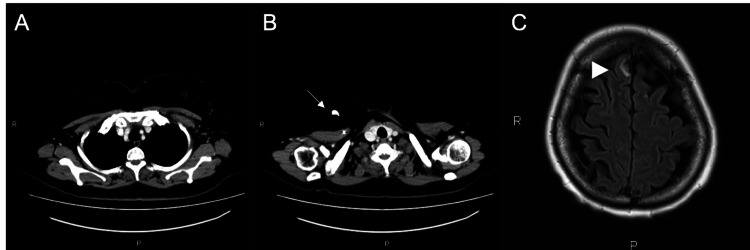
Imaging findings after six cycles of TPD CT scan (A, B) and MRI of the brain (C). The white arrow points to the central venous port. The arrowhead points to the largest of the multiple brain metastases. TPD: Trastuzumab + pertuzumab + docetaxel; CT: Computed tomography; MRI: Magnetic resonance imaging
